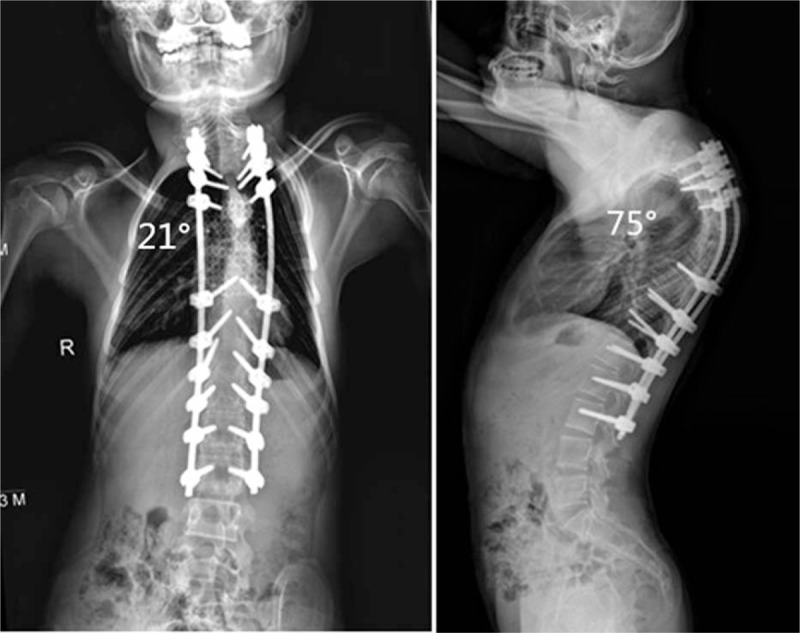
At the final follow-up, long-standing scoliosis radiographs revealed the coronal main curve was corrected to 21° and sagittal kyphosis curve to 75°.
